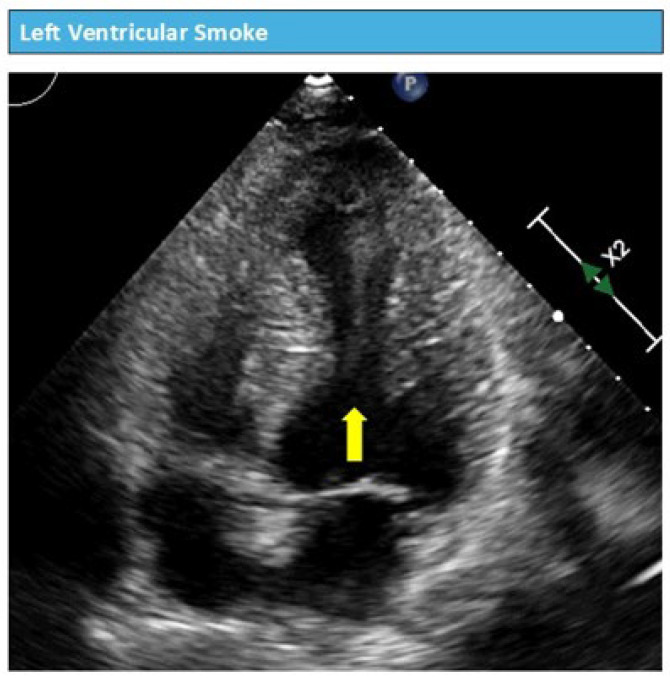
Echocardiographic evaluation of Blood stasis. Spontaneous echocardiographic contrast (smoke) is caused by increased ultrasound backscatter due to the agglomeration of cellular blood components in low-flow states. Yellow arrow: spontaneous echocardiographic contrast (‘smoke’) within the left ventricular cavity, indicative of blood stasis and increased thromboembolic risk.
